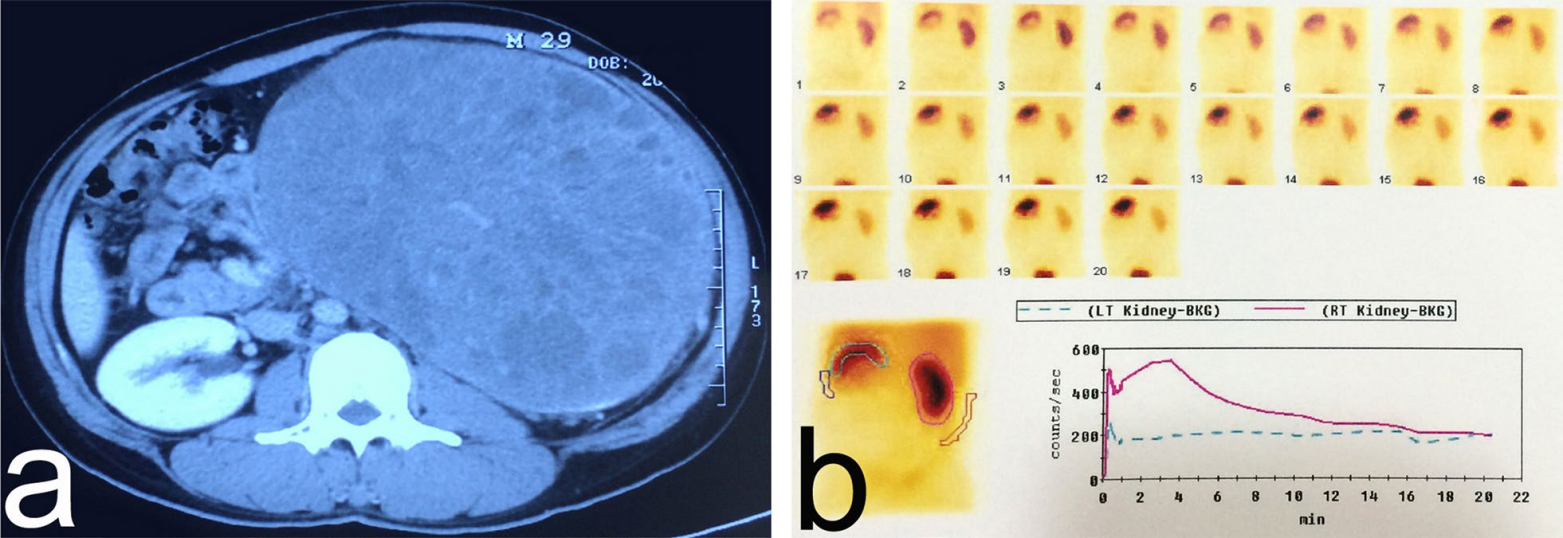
Computed tomography and emission computed tomography of a 29-year-old man with a giant abdominal mass. a Computed tomography of the abdomen on May 6, 2015 before the surgery demonstrates a giant (22 cm × 16 cm) solid mass in the left kidney without necrosis or calcifications. Heterogeneous enhancement can be seen during the arterial phase, and normal renal tissues are seriously squeezed. b Emission computed tomography reveals mild-to-moderate damage of the left kidney and normal function of the right kidney
